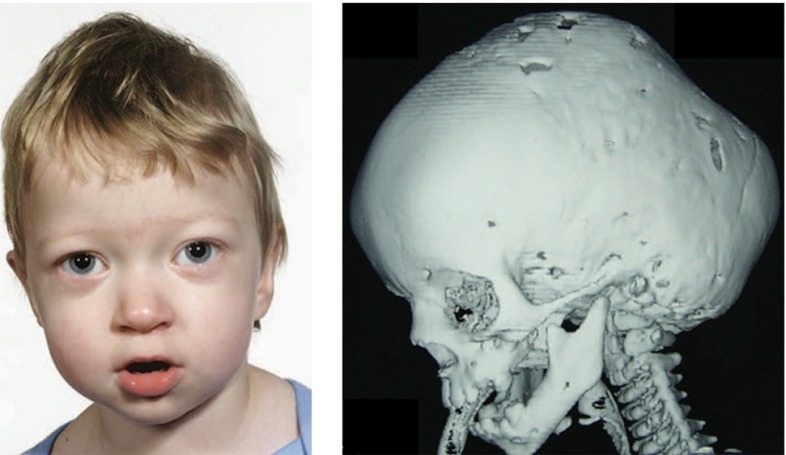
Clinical photograph and computed tomographic scan of a pediatric patient a year after modified strip craniectomy for sagittal synostosis, demonstrating vertex bulge and bicoronal synostosis (Marucci et al. 2008)
